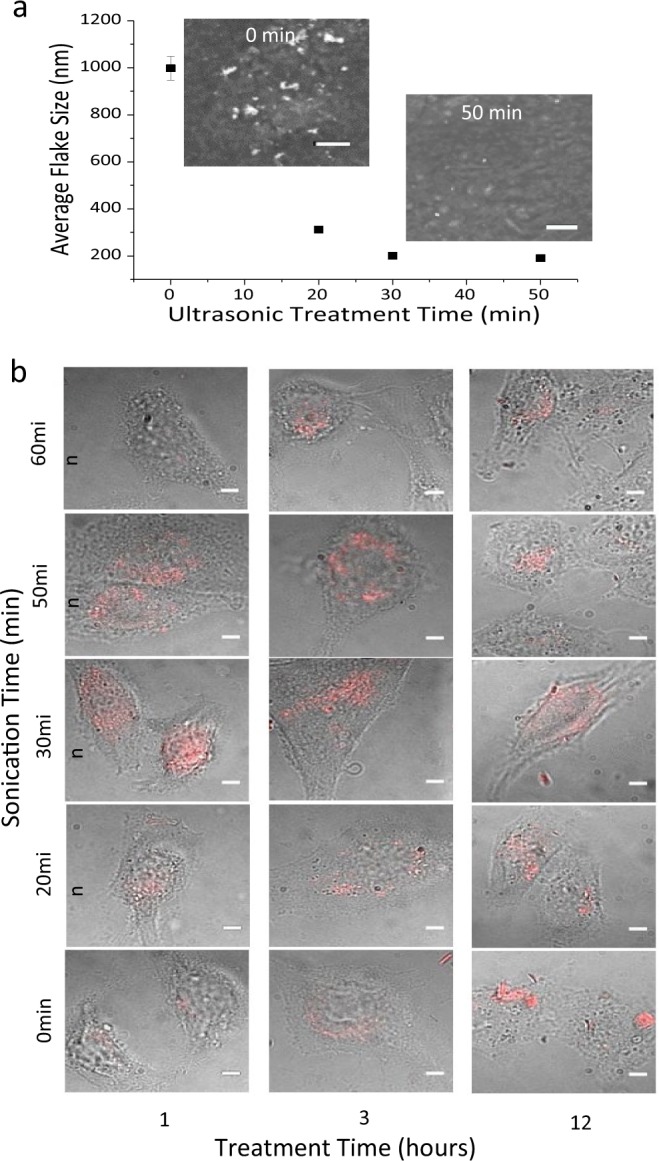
(a) Mean Flake Size vs. ultrasonic treatment time from SEM analysis. Insets are SEM images of GO flakes subject to 0 min and 50 min ultrasonic treatment. (b) Image matrix of GO emission in HeLa cells with varying ultrasonic treatment time (vertical) vs. transfection time (horizontal). Scale bar length is 5 μm.
